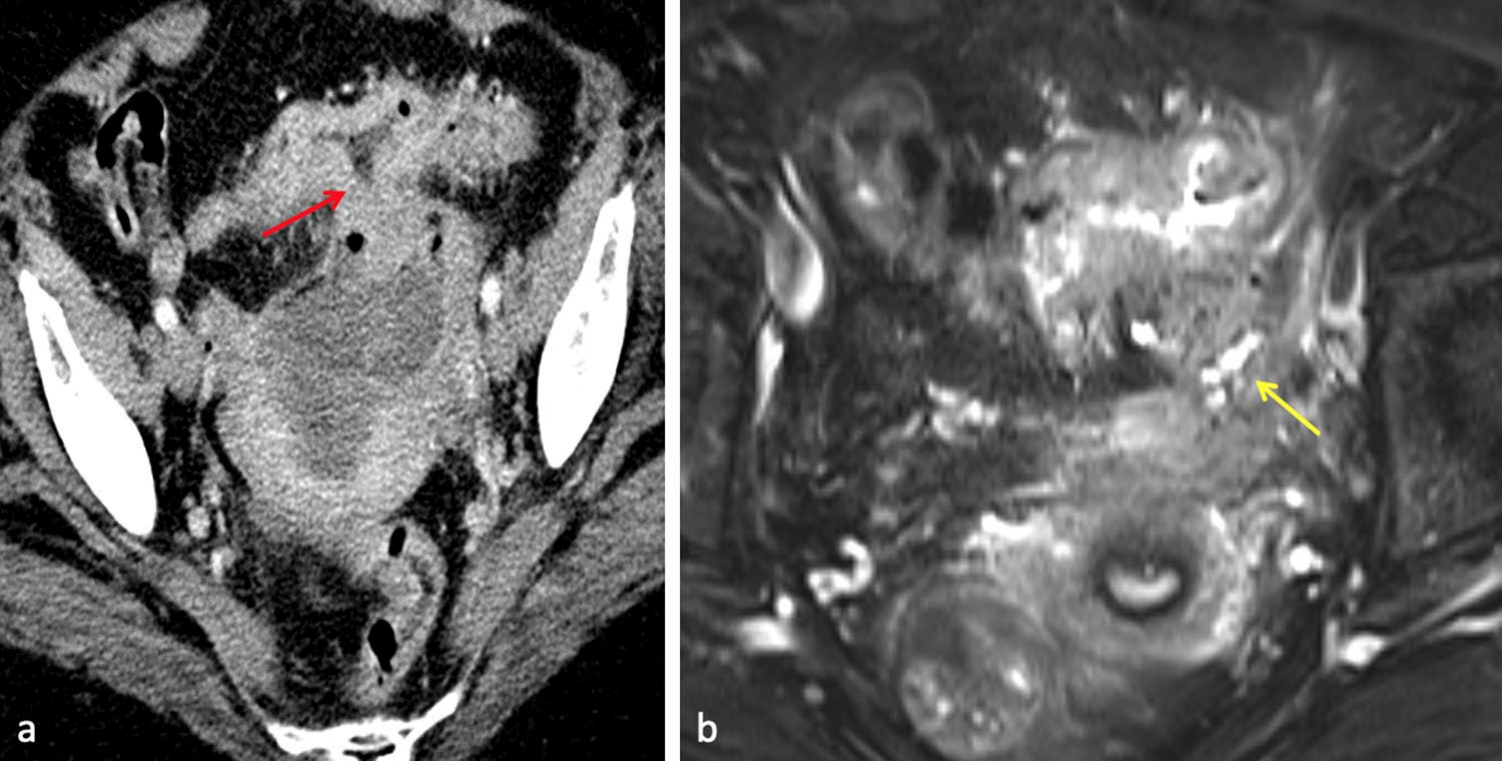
61-year-old female presenting with abdominal pain, fevers, and feculent vaginal drainage. Contrast-enhanced CT a demonstrates sigmoid wall thickening and adjacent stranding consistent with acute diverticulitis. Associated abscess and soft tissue tract with locules of gas between the inflamed sigmoid colon and uterine fundus (red arrow) is concerning for colouterine fistula. Axial T2-weighted fat saturated MR b demonstrates a hyperintense fistulous tract (yellow arrow). The patient improved with IV antibiotics
